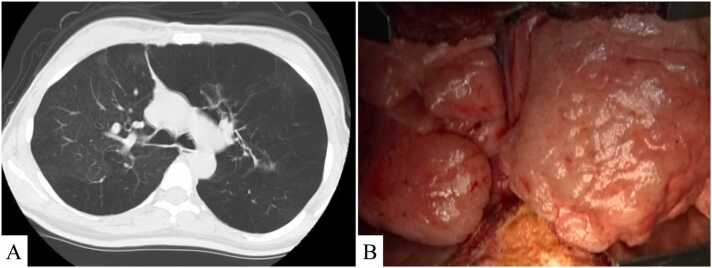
Computed tomography revealed emphysema and lung hyperinflation before transplantation (A). Intraoperatively, the recipient's lungs lacked elasticity (B).
